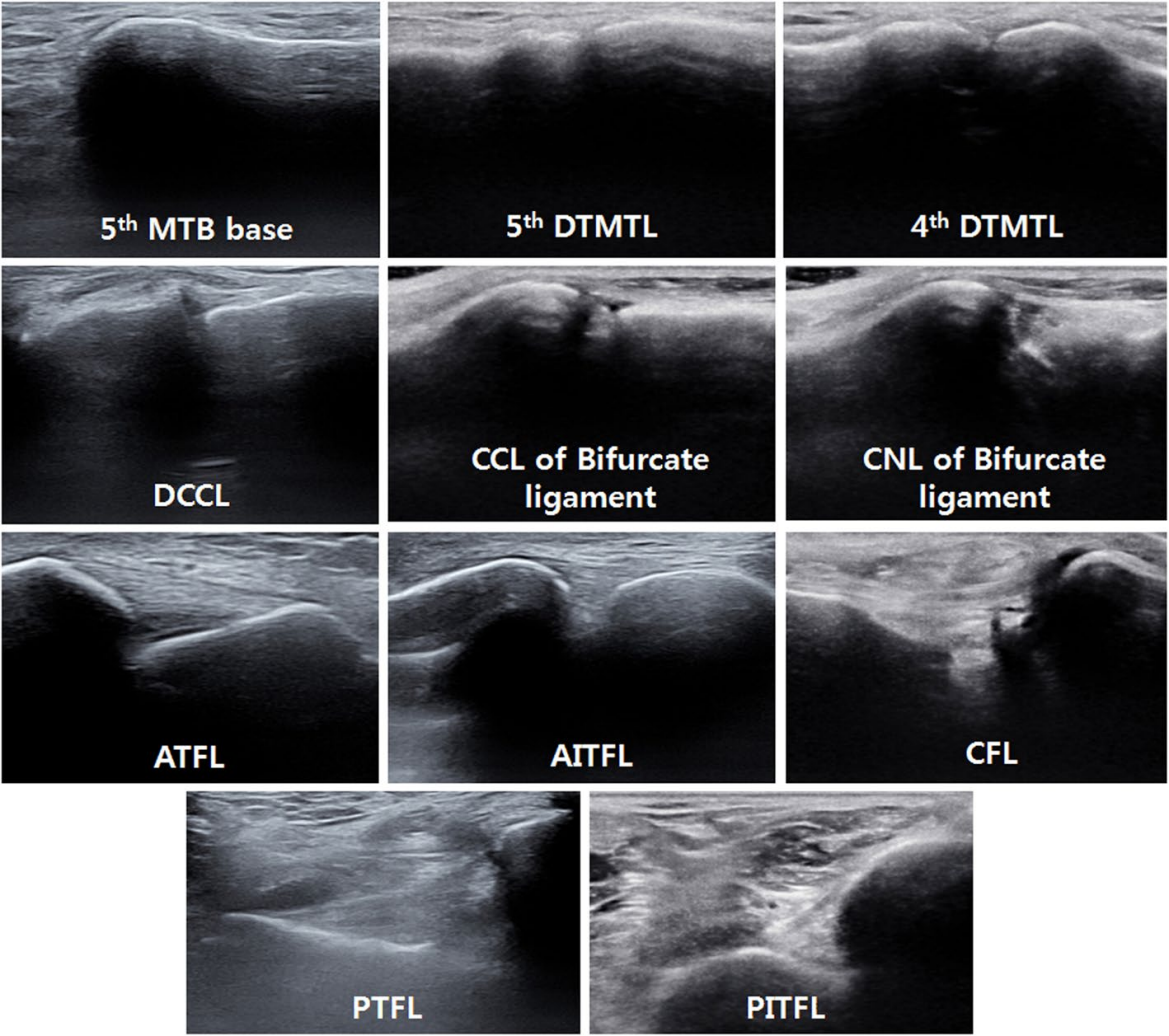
Normal ultrasound findings of each ligament. 5th MTB, fifth metatarsal bone; 5^th^ and 4^th^ DTMTLs, fifth and fourth dorsal tarsometatarsal ligaments; DCCL, dorsal calcaneocuboid ligament; CCL, calcaneocuboid ligament; CNL, calcaneonavicular ligament; ATFL, anterior talofibular ligament; AITFL, anterior inferior tibiofibular ligament; CFL, calcaneofibular ligament; PTFL, posterior talofibular ligament; PITFL, posterior inferior tibiofibular ligament
